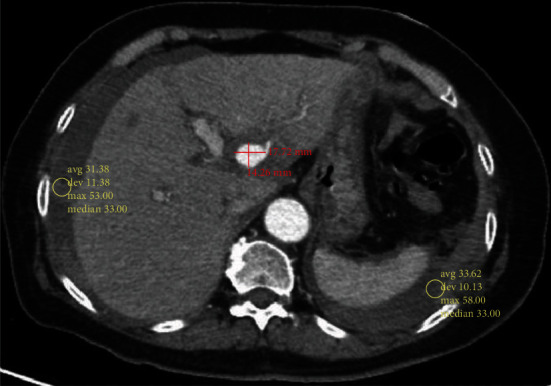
Axial view of CT abdomen and pelvis with IV contrast. There is a pseudoaneurysm from the left hepatic artery going to the segment II, measuring approximately 1.4 cm × 1.8 cm × 1.9 cm. Also, pertinent for an extensive hemoperitoneum particularly around the liver, around the spleen, through right and left paracolic gutter, and in the pelvis.
